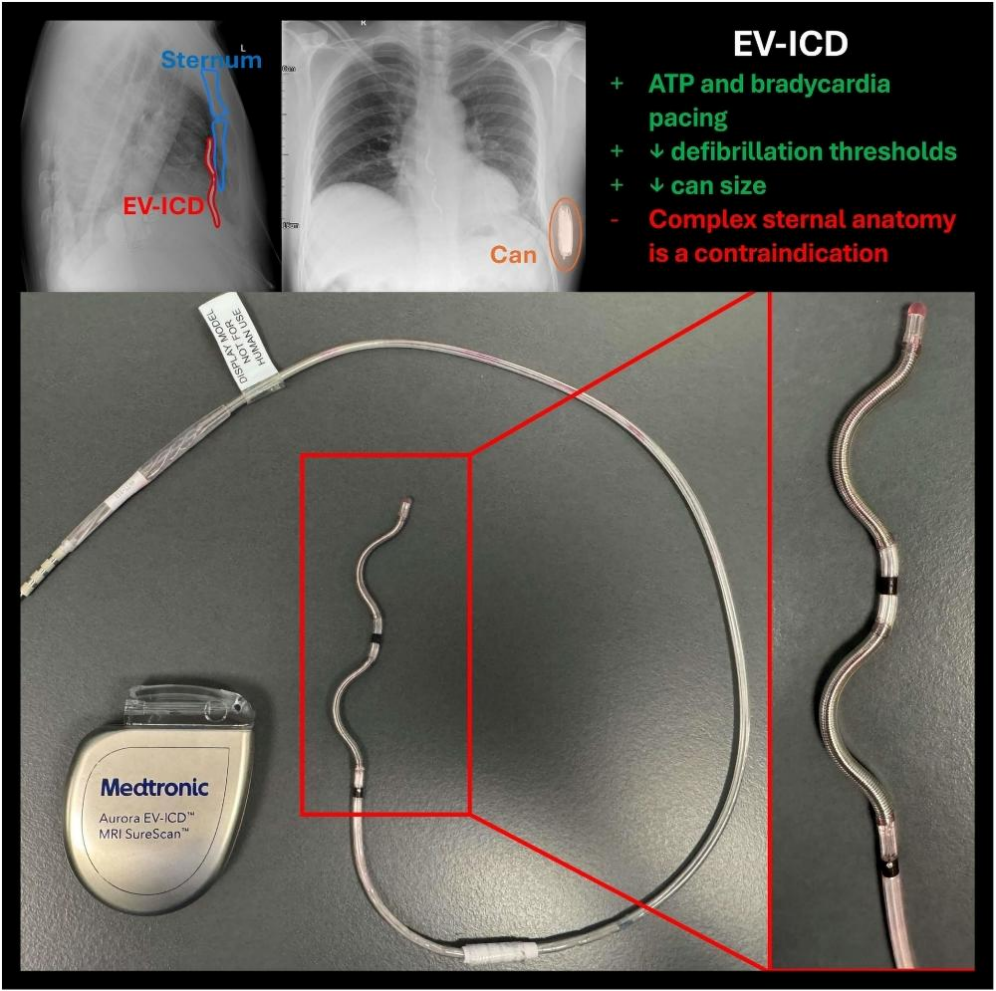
(*A*) Extravascular ICD lead in relation to the sternum in pectus excavatum (see antero-posterior and lateral chest X-ray views). (*B*) Dummy device (EV-ICD) used for patient education and pre-operative planning; of note is the reduced can size (64 × 51 × 13 mm) compared with prior SC-ICD (83.1 × 69.1 × 12.7 mm) and novel lead design.
